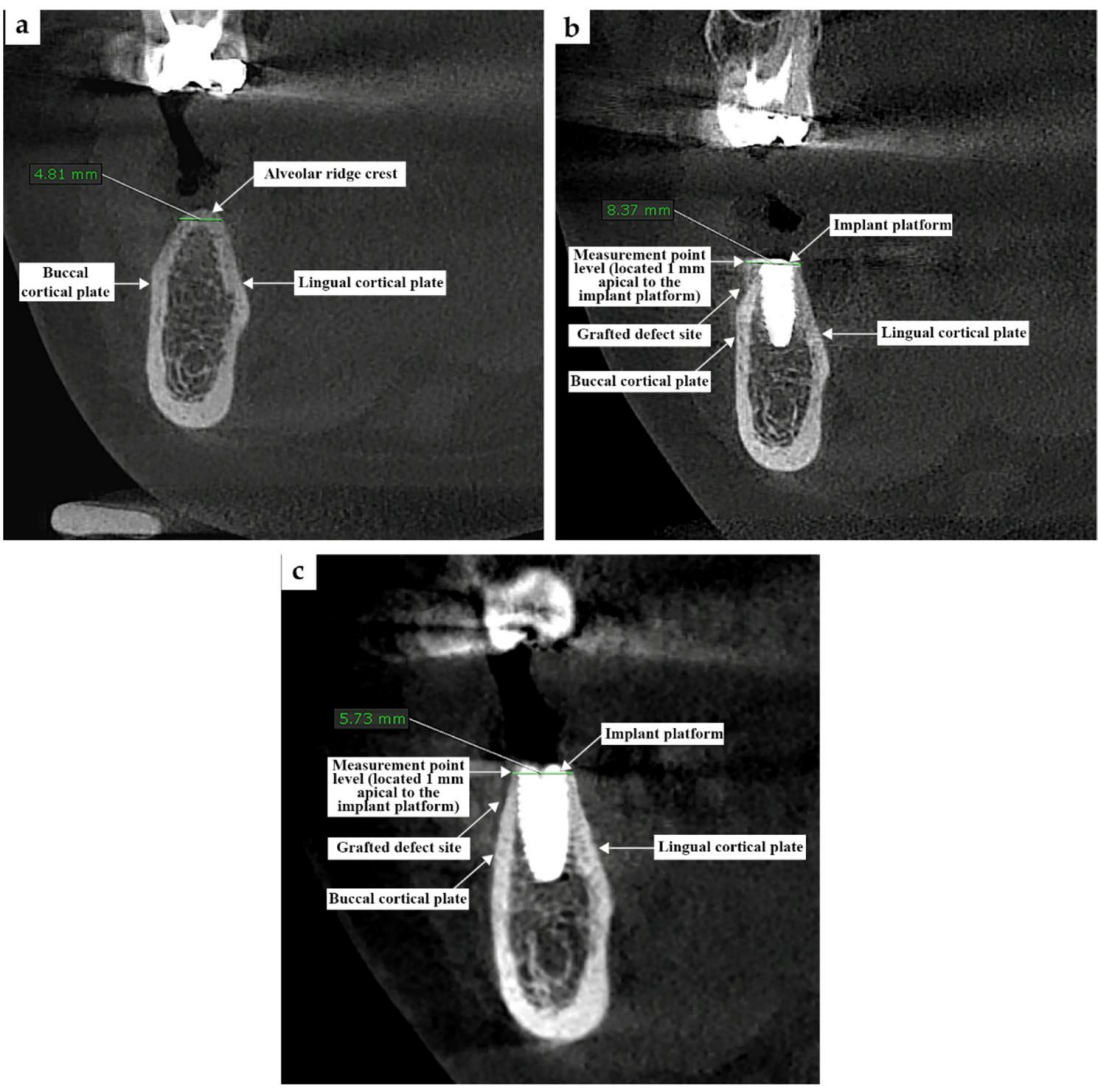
CBCT measure net: (a) pre-operative cone beam computed tomography (CBCT), (b) Immediate CBCT, (c) CBCT after six months
